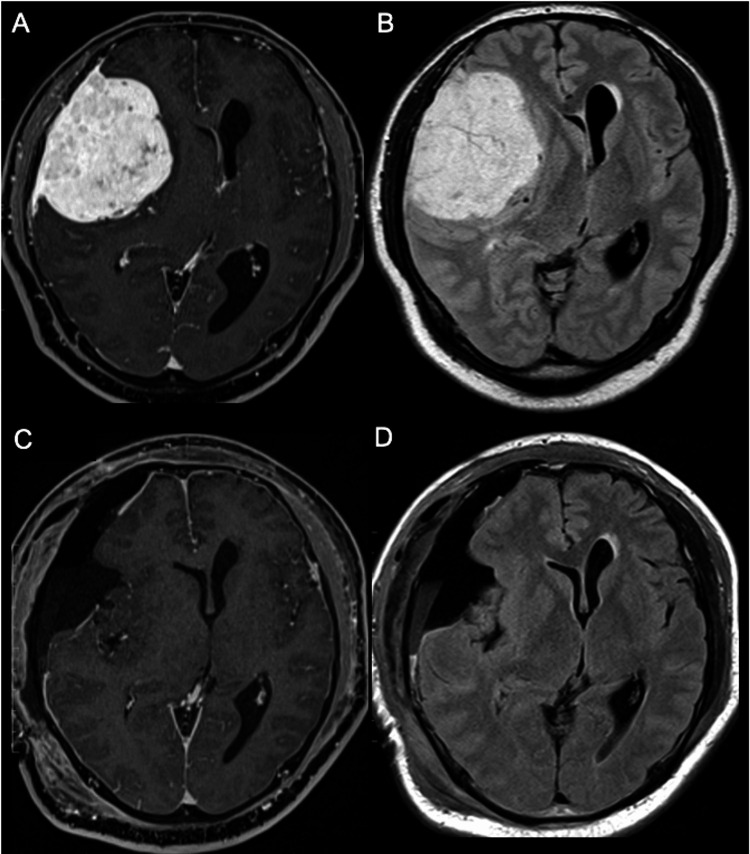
Pre- and postoperative magnetic resonance images of Case 3. (A) Preoperative Gd enhanced axial T1WI. (B) Preoperative axial FLAIR image. (C) Postoperative Gd enhanced axial T1WI. (D) Postoperative axial FLAIR image. Gd: gadolinium; T1WI: T1-weighted image; FLAIR: fluid-attenuated inversion recovery
